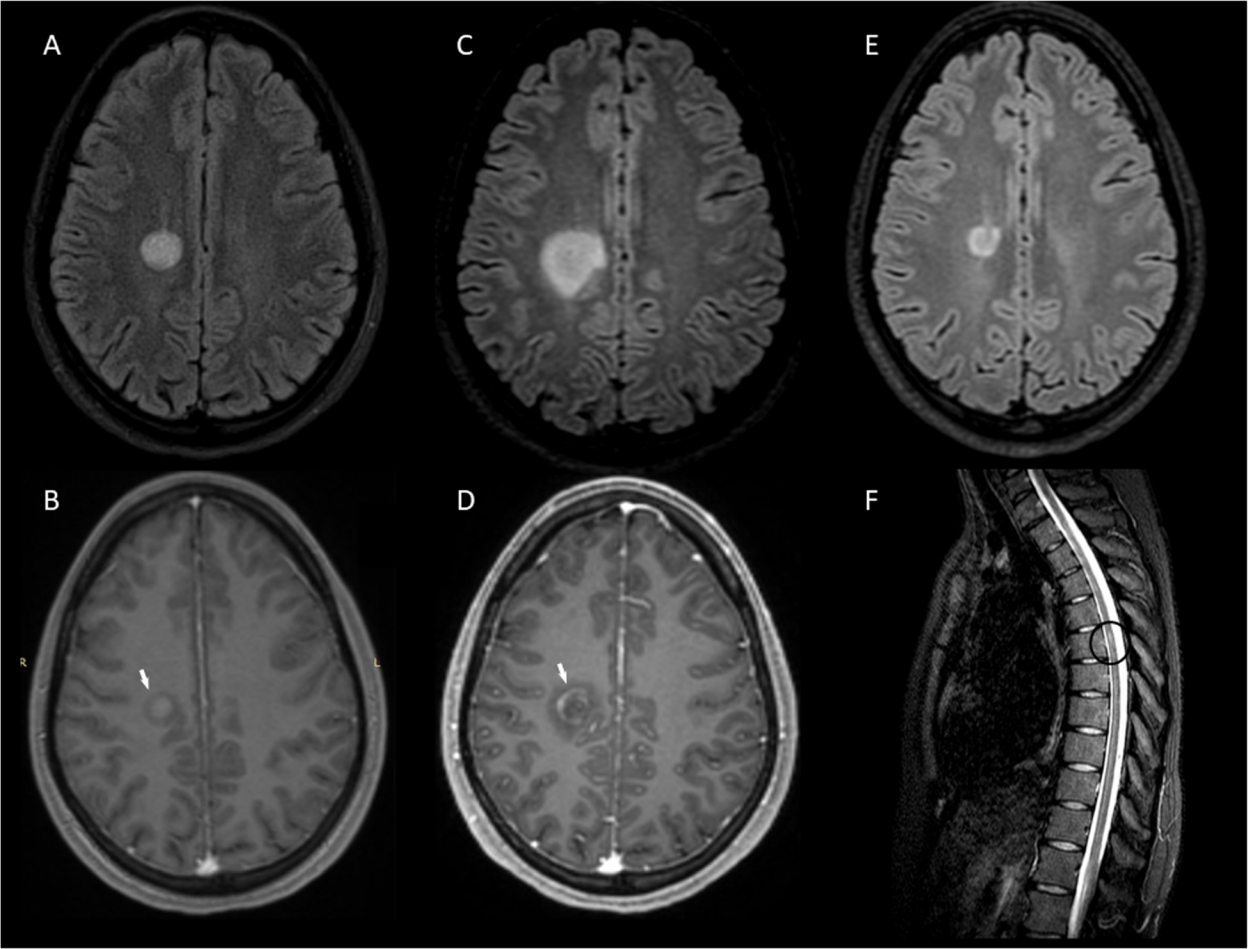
MRI of Case 7 before and after treatment with rituximab. A BCS-type lesion in the right centrum semiovale (A) with peripheral almost complete Gd + at outer layer (B arrow) at symptom onset. Follow-up MRI at 1 month, after corticosteroid treatment, showing enlargement of the BCS-type lesion (C) with Gd + (D arrow) and one lesion at Th6 level (F circle). BCS-type lesion (E) 4 months from the first rituximab course, 5 months from BCS onset. a, c, e: 3D FLAIR images. b, d: 3D T1-weighted contrast-enhanced images. f: STIR image. BCS: Baló’s concentric sclerosis, Gd+: gadolinium enhancement
